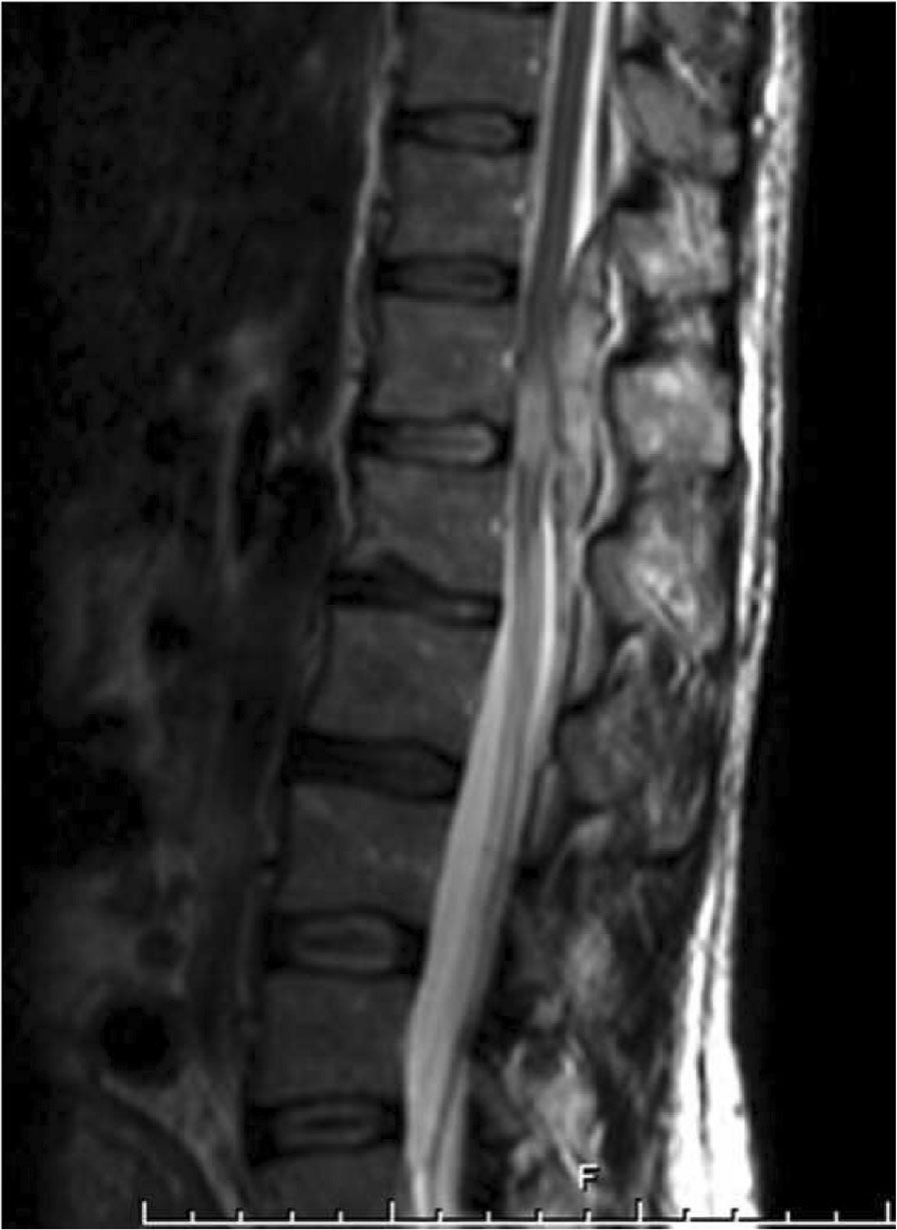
Sagittal T2-weighted MRI shows hyperintense collection in the posterior spinal epidural space at level D12-L1 and spinal cord signal alteration
